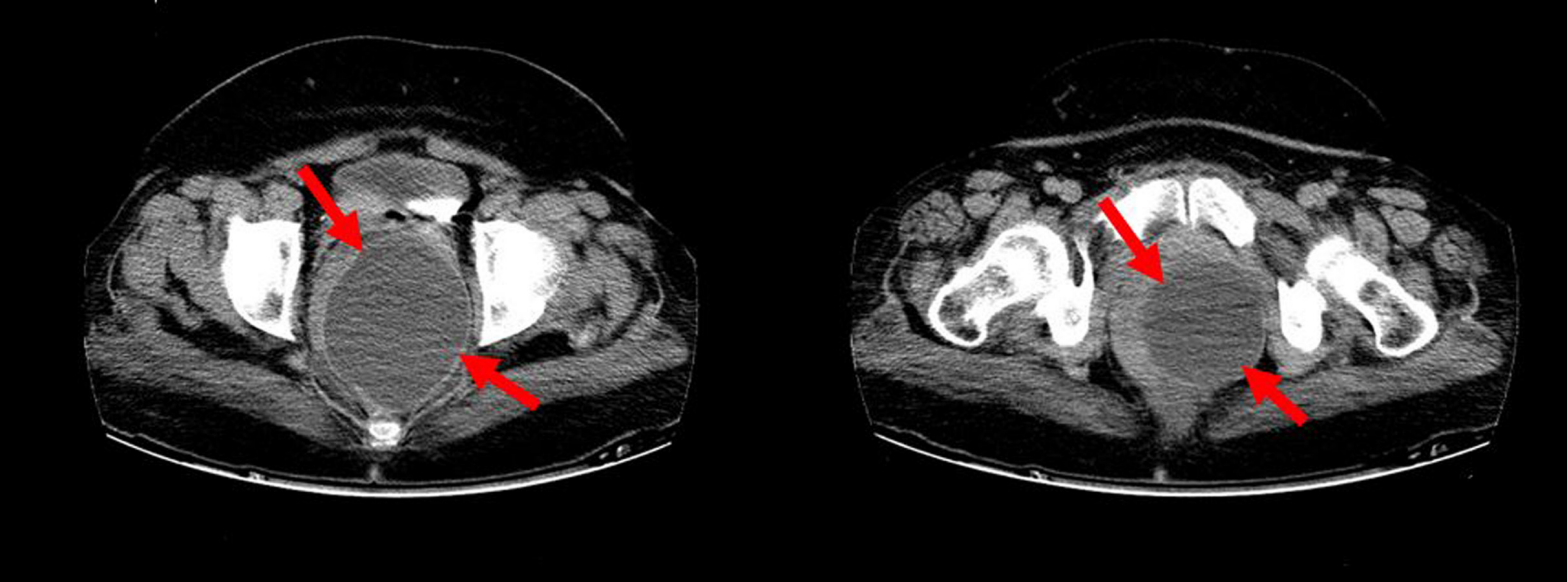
Contrast computed tomography reveals a hypodense cystic lesion measuring about 99*93 mm in the left retro‐rectal region (arrowhead) without contrast enhancement, causing a rectal deviation to the right side.
